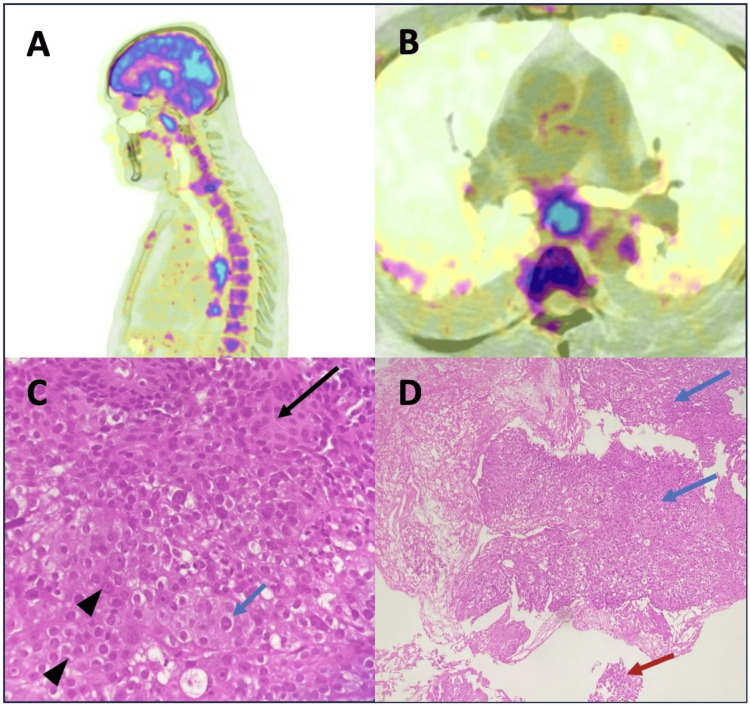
PET/CT and biopsy findings (A, B) PET/CT whole body showing malignant growth in the thoracic oesophagus; (C) Pleomorphic cells with abundant eosinophilic cytoplasm (black arrow heads), with large vesicular nuclei and prominent nucleoli (blue arrow), with individual cell keratinisation (black arrow); (D) Infiltrating tumor composed of atypical cells arranged in sheets and nests (blue arrow), with high grade dysplasia in the adjacent epithelium (red arrow).
